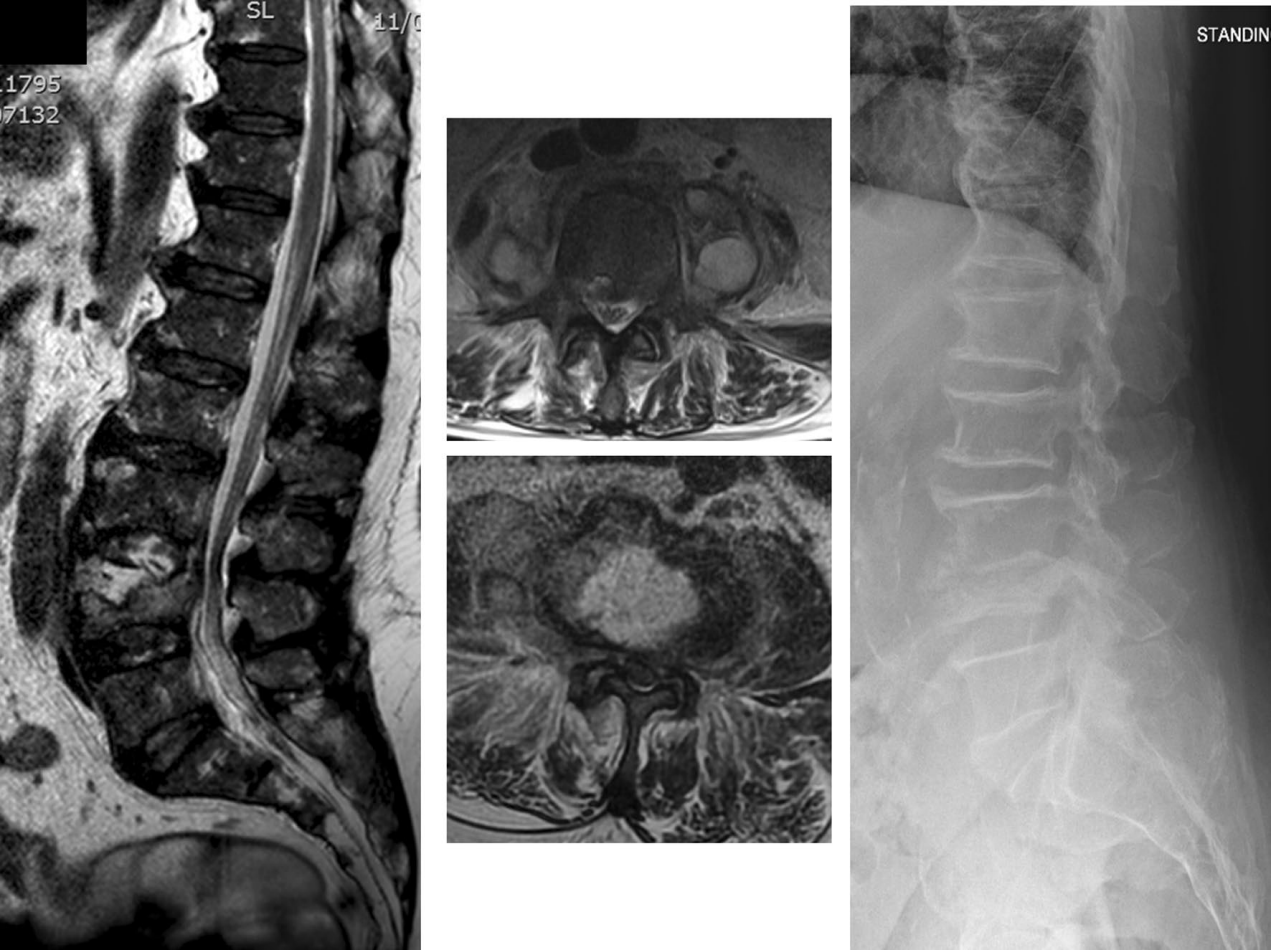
A 75-year-old patient with pyogenic spondylodiscitis at L3/L4. *S. aureus* had been identified by blood cultures 10 days earlier and IV antibiotic therapy was started soon afterwards. After 10 days of IV therapy, the patient was still complaining of significant pain with incomplete improvement of the inflammatory markers (CPR 135 mg/L). (Left panel) MRI sagittal view of the involved disc space; (middle panel) axial view at the level of the L3/L4 disc space and the L4 vertebral body showing a significant disc abscess and bilateral psoas abscesses. (Right panel) standing X-ray of the lumbar spine showing resolution of the infection and fusion of the involved segment 8 months after the end of treatment
